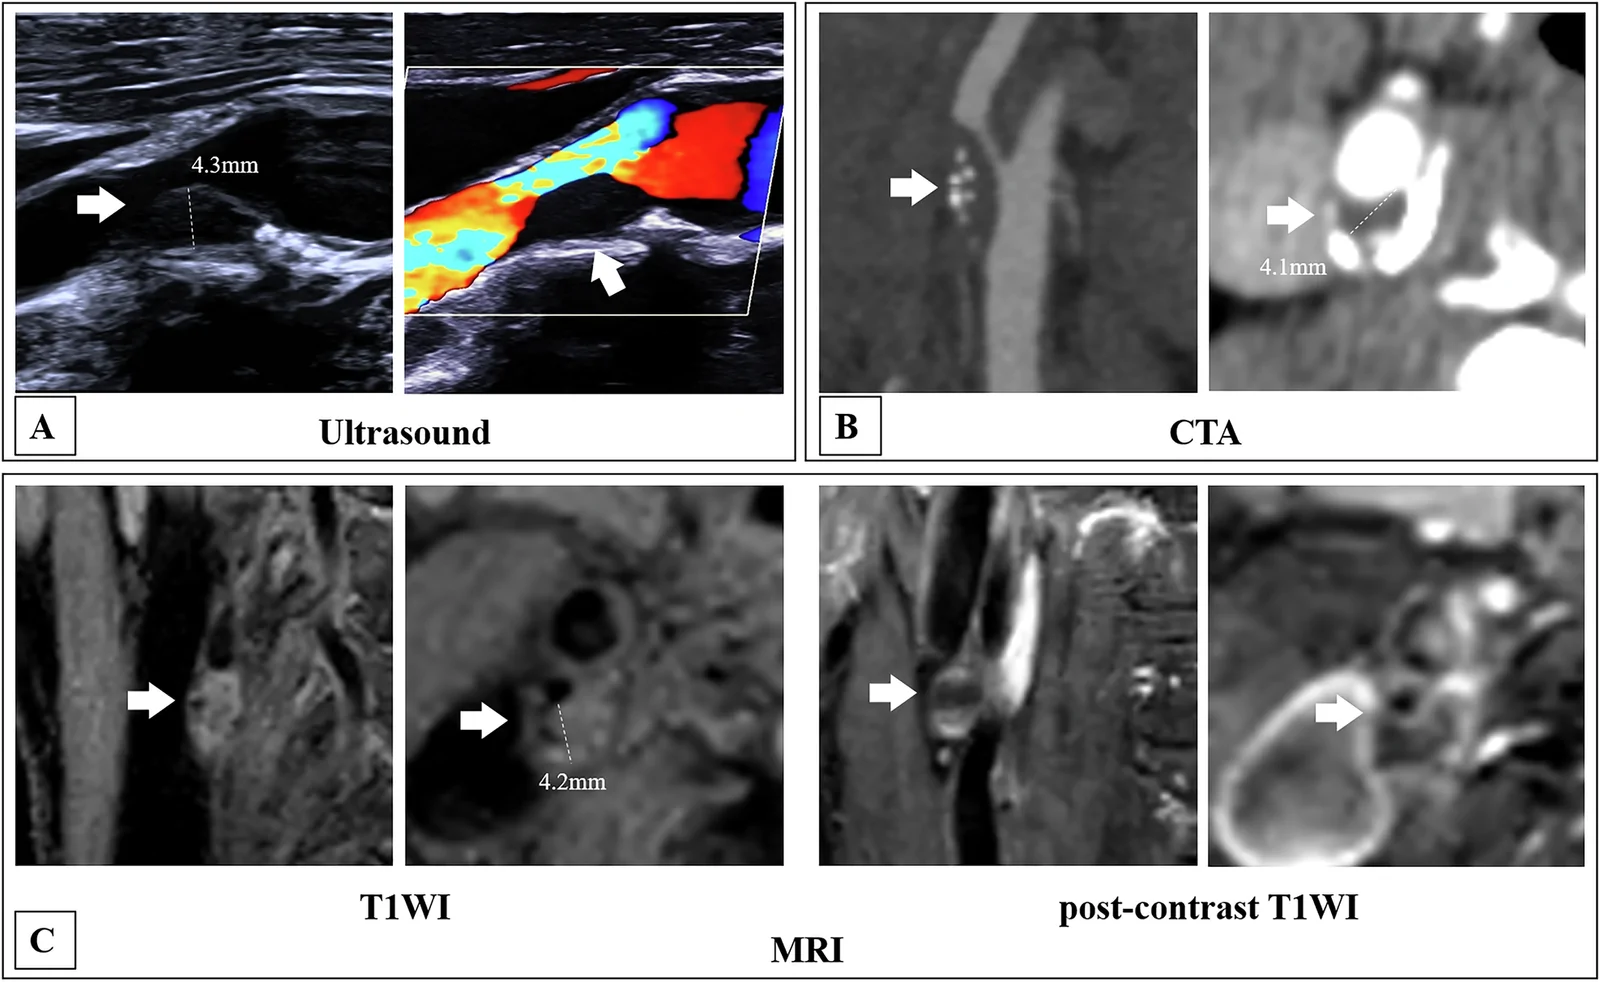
A 70-year-old male with severe stenosis of the right internal carotid artery, classified as Plaque-RADS score 4. A US reveals an eccentric plaque (arrow) in the proximal right internal carotid artery, demonstrating a maximal wall thickness of 4.3 mm, a gray-scale median of 20, and a juxtaluminal black area. B CTA identifies the plaque (arrow) as a mixed type, featuring a hypodense core with a measurement of 19 HU and maximal wall thickness of 4.1 mm. C MRI demonstrates that the plaque (arrow) has moderately high signal intensity on T1WI and shows no enhancement on post-contrast T1WI, findings which are consistent with IPH
